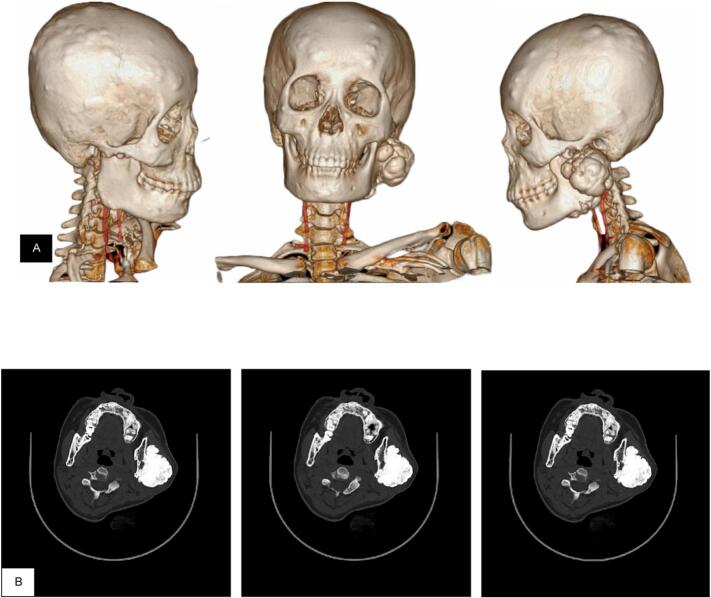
A. MSCT of pre-hemi-mandibulectomy: shows multiple rounded lesions, firm borders, and regular edges in bilateral parietal, temporal bilateral, and frontal bilateral. Multiple lesions of the mandibular region with 5.6 × 6.0 × 4.5 cm in size, B. CT cross-sectional image shows lesions in left mandibular region. The lesion is arising on the surface of the bone and homogenously dense.
